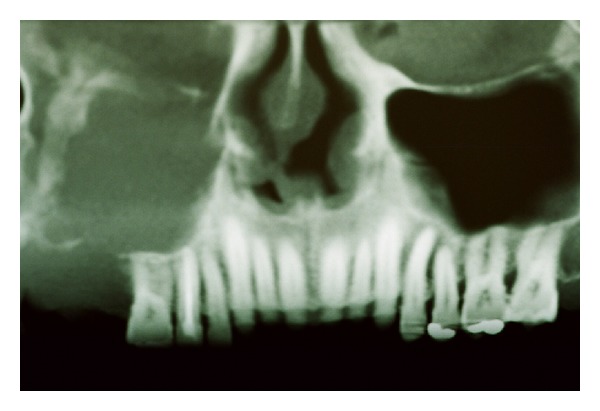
Cone beam computed tomography scan showing a sinonasal mass causing opacification of the right maxillary sinus with erosion of the orbital floor, lateral nasal wall, and maxillary sinus floor.
